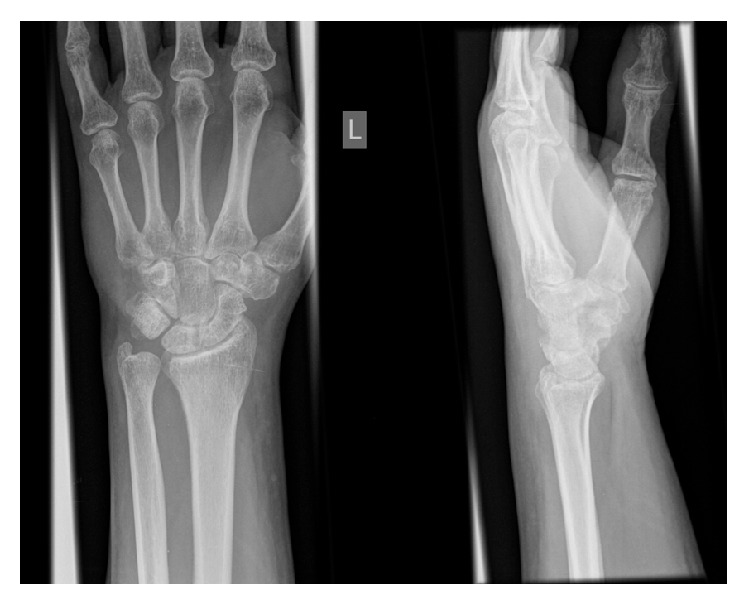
Radiographs at 3 months.
